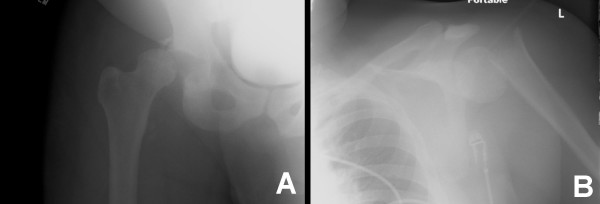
Radiographs of initial acetabular fracture dislocation and initial proximal humerus fracture.
